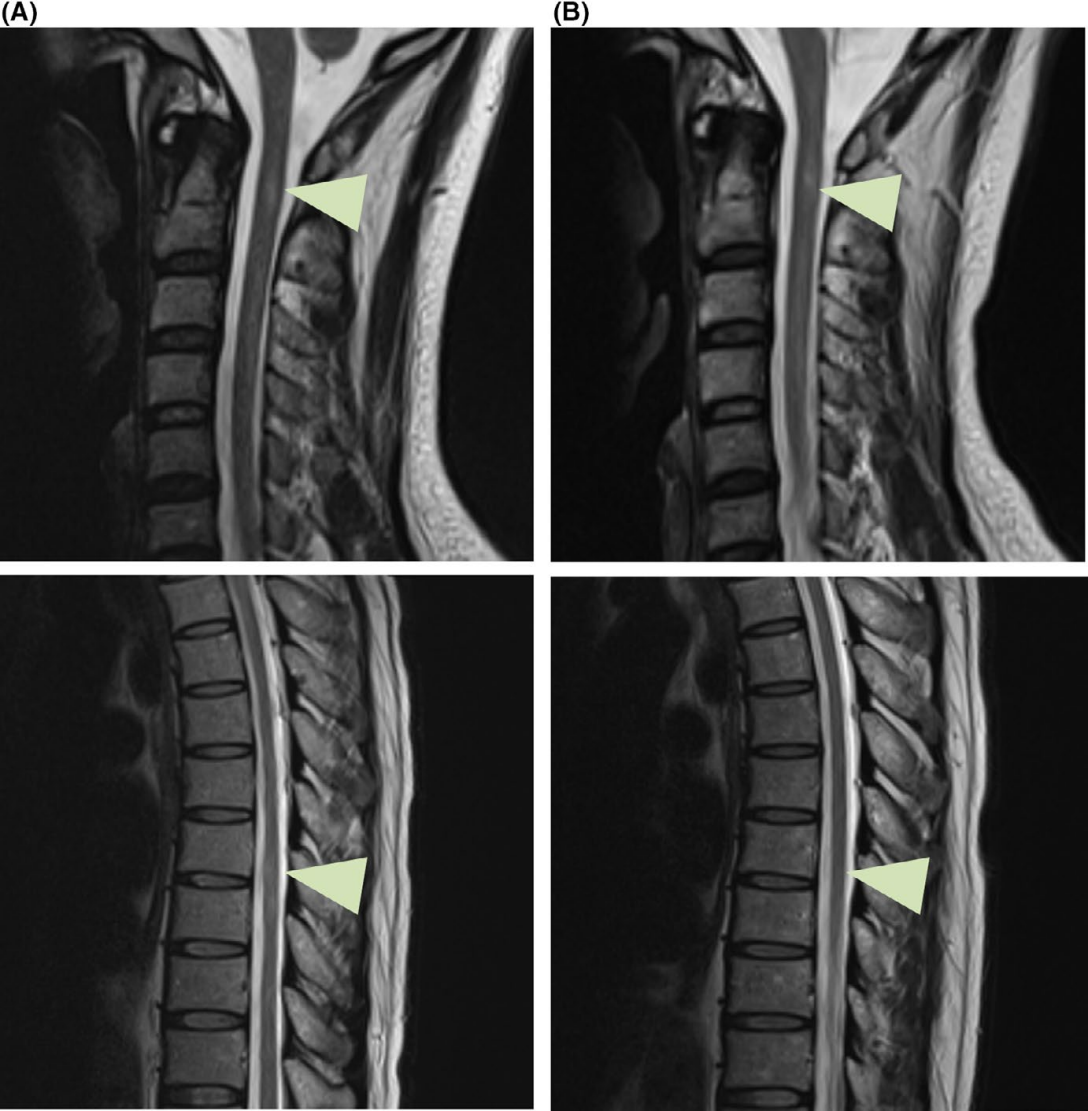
Myeleterosis. MRI scan of spinal cord showed hyperintensity in C2 and T8/9 level (A). A follow‐up MRI scan showed the abnormality of spinal cord had narrowed (B)
